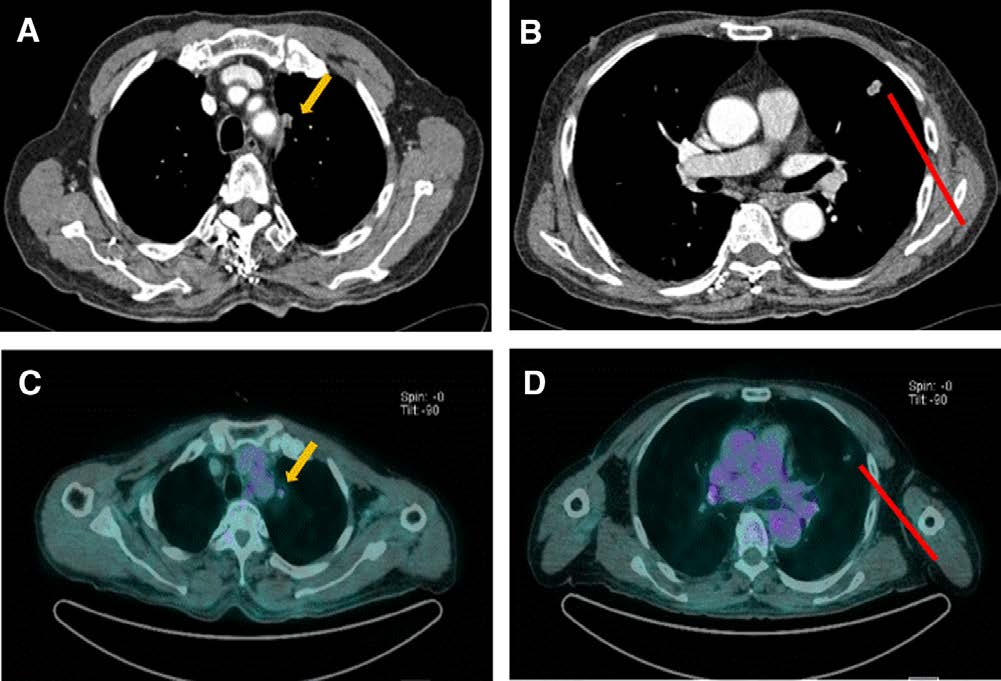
Contrast-enhanced Chest CT (A, B) and PET-CT (C, D). (A, B) Chest CT revealed 9.5 mm sized speculated nodule in left upper anterior segment (yellow arrow) and 15.2 mm sized irregular nodule in left lingular segment (red row). (C, D) PET-CT showed mild FDG-uptake in respectively. CT = computed tomography, FDG = 18-Fluoro-deoxyglucose, PET = Positron emission tomography.
